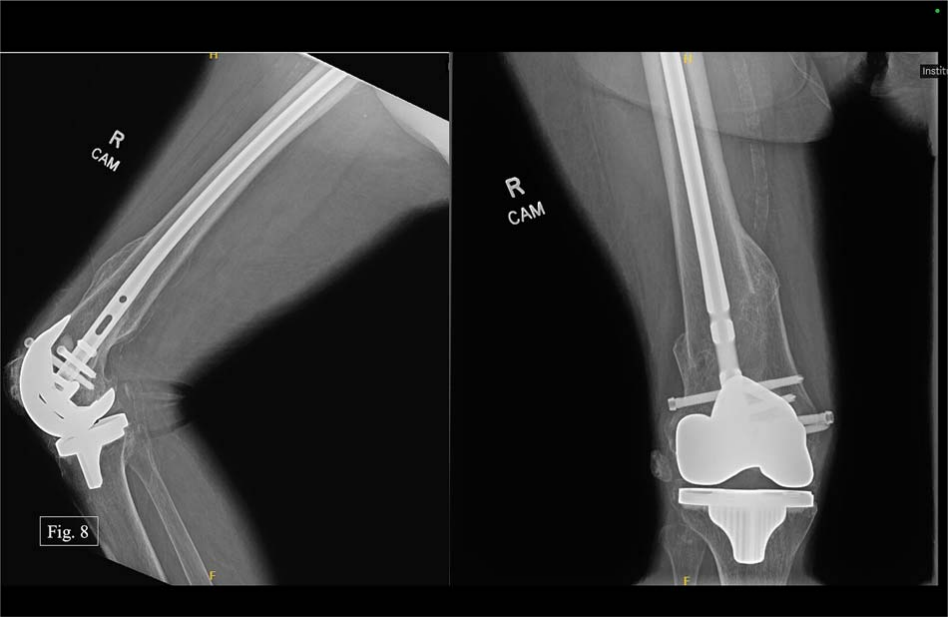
Right, 17 month postoperative AP and lateral X-rays.
